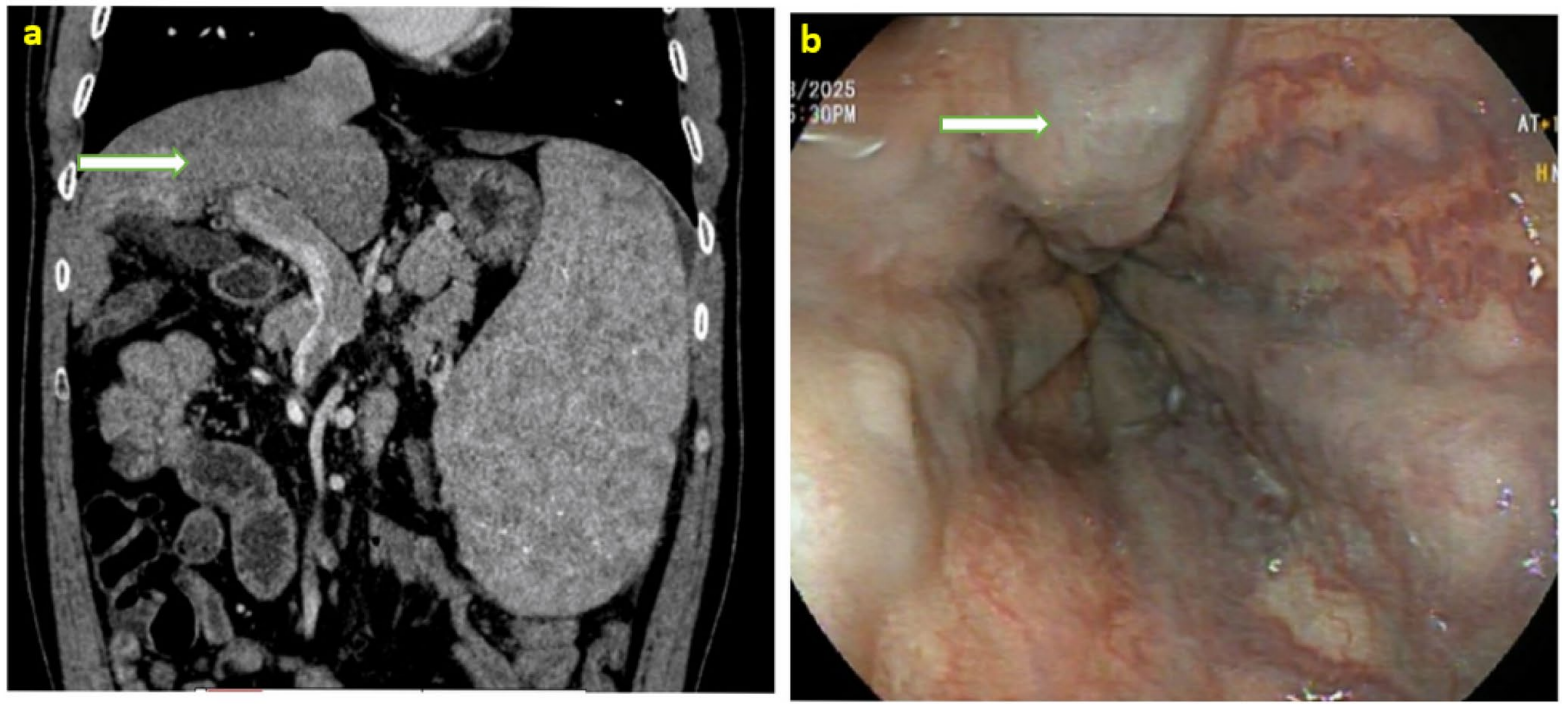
(a) CT abdomen (Arterial phase in coronal section with slice thickness of 1 mm) showing cirrhotic liver with dilated portal vein and splenomegaly. (b) Upper gastrointestinal endoscopy showing high‐risk esophageal varices (Grade III).
